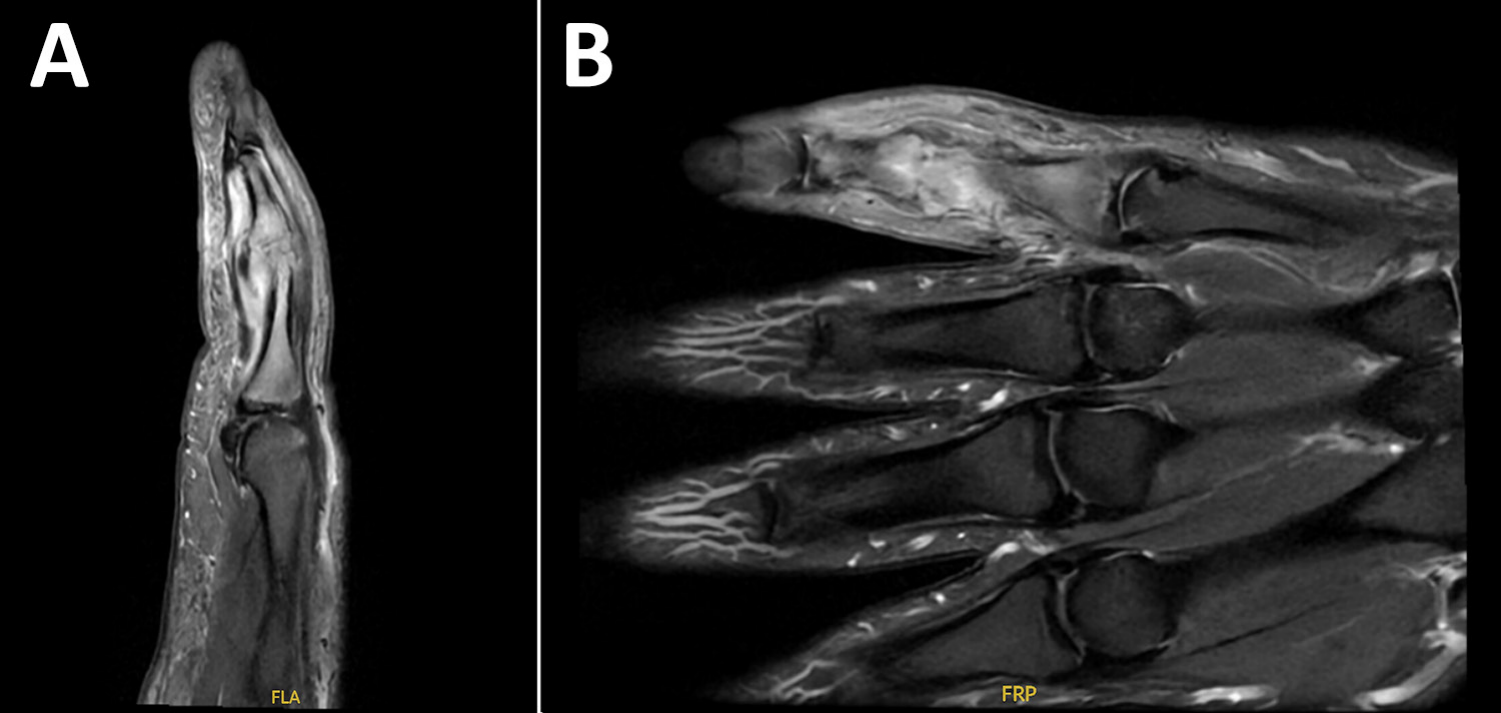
Magnetic resonance imaging in case of septic arthritis and osteomyelitis in finger caused by *Mycoplasma phocimorsus* from brown bear (*Ursus arctos*), Alaska, 2024. Left fifth finger sagittal (A) and coronal (B) images demonstrate edema of the proximal and middle phalanges on short tau inversion recovery sequences, suggestive of osteomyelitis.
